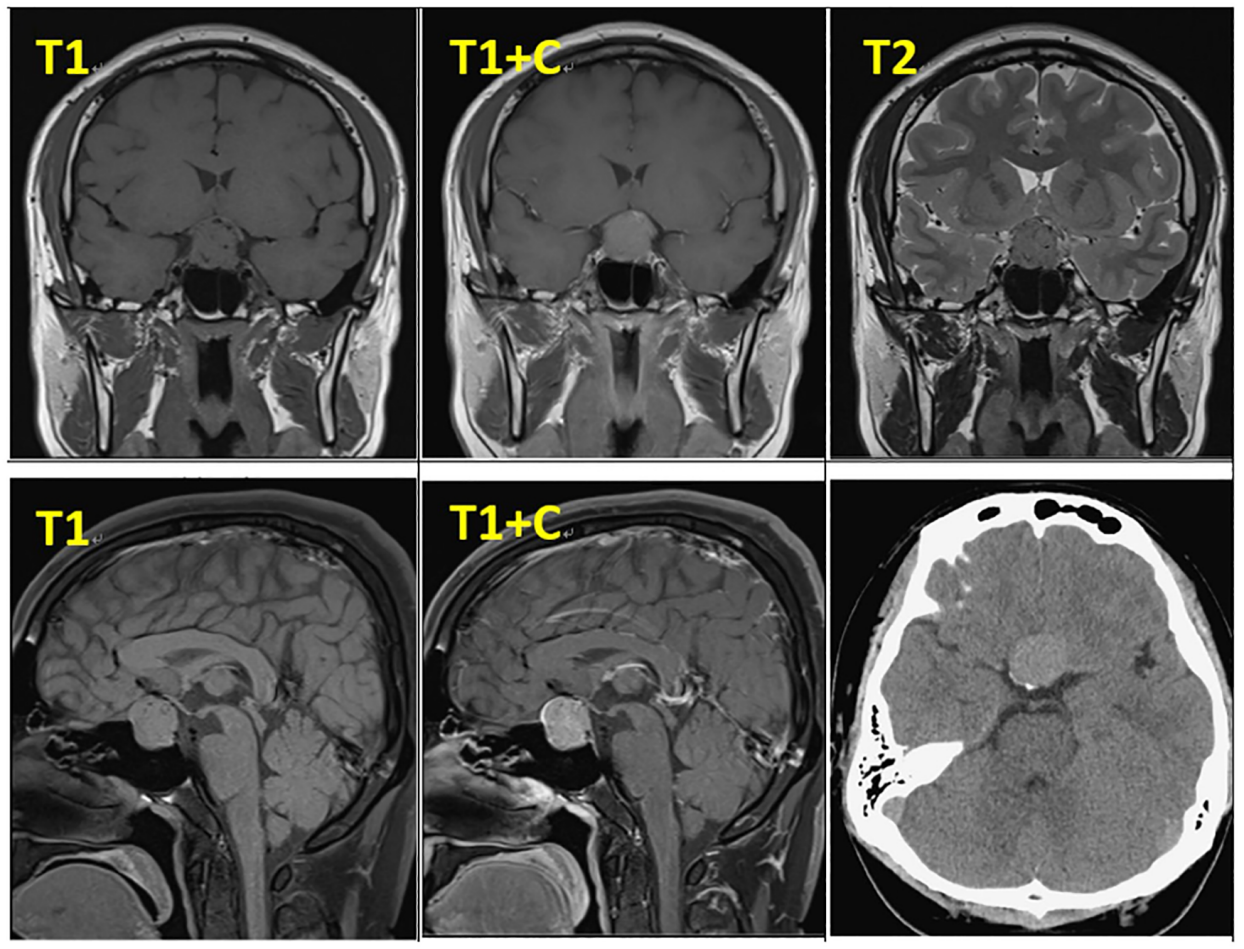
Pre-operative MRI and CT.
